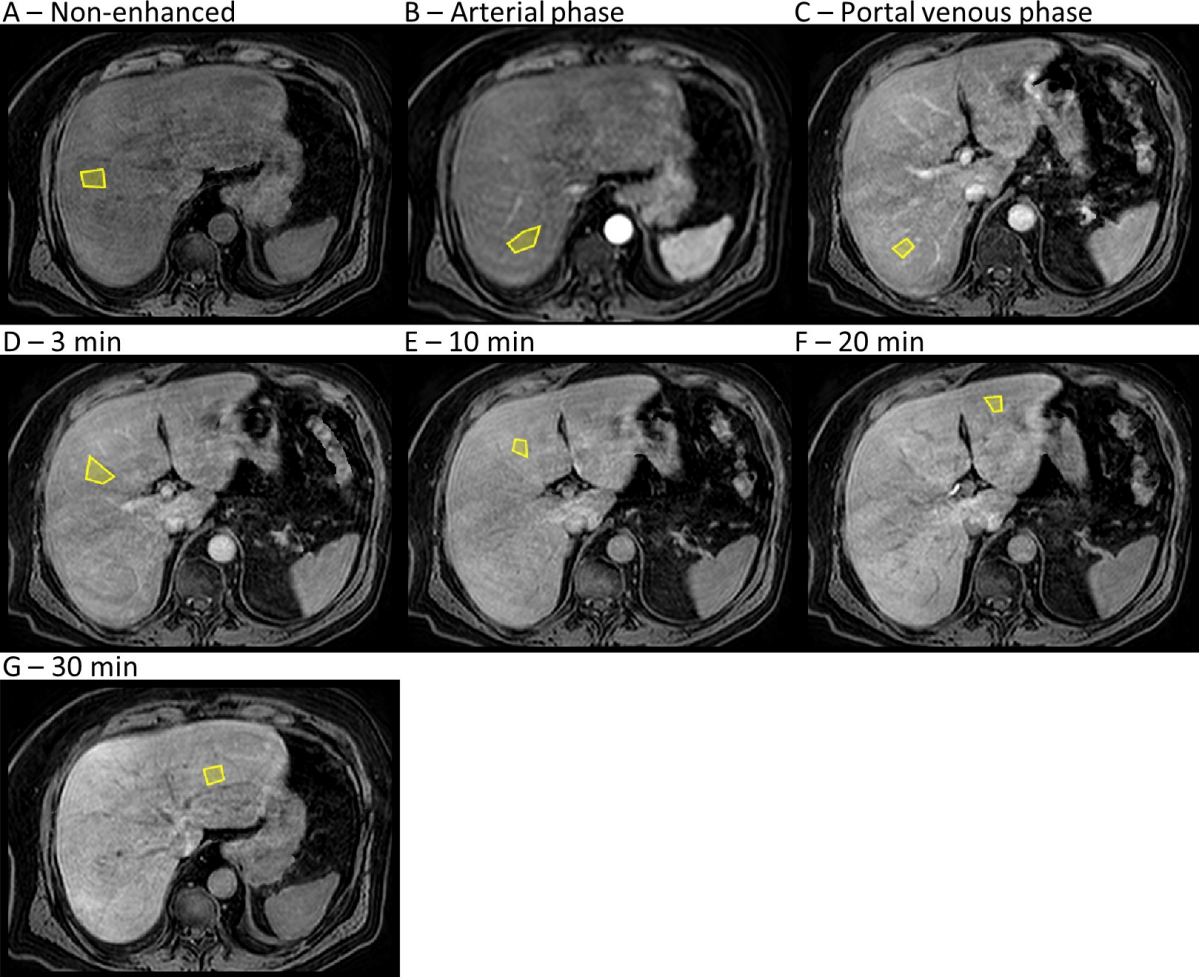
Gadoxetate MRI time series of the liver. (A-G) Representative placement of seven regions of interest (yellow polygons) within the liver, of which four (A-D) were placed in the right liver lobe and three (E-G) were placed in the left liver lobe. This set shows an entire time series in a single patient, from before gadoxetate injection (A) to 30 min after gadoxetate injection (G). The arterial phase (B) typically occurs 30 s after gadoxetate injection, and the portal-venous phase (C) typically occurs 1 min after gadoxetate injection.
